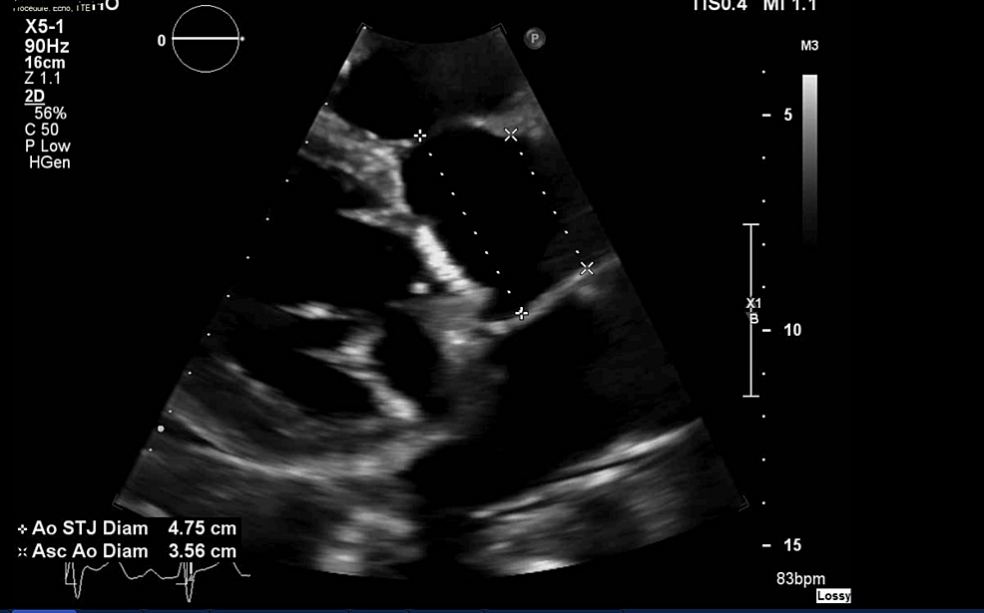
TTE showing a dilated aortic root, measuring 4.75 cm at the sinotubular junction TTE: transthoracic echocardiogram
